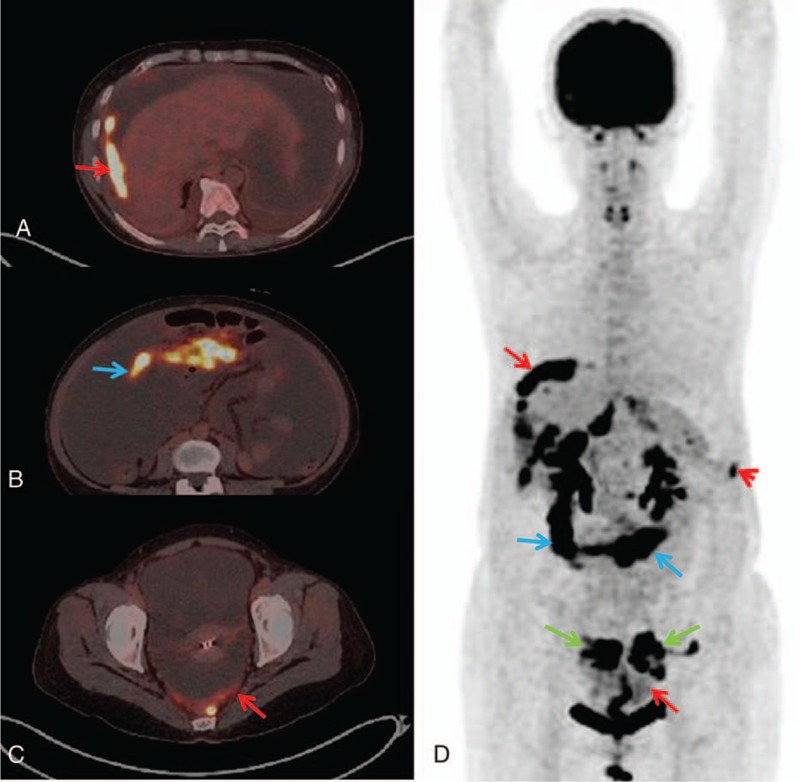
A 46-year-old woman with peritoneal carcinomatosis from ovarian cancer. Dominant distribution in the pelvic and right subdiaphragmatic region of the parietal peritoneum (the susceptible area for peritoneal implantation, SAPI) (SAPI distribution): clustered ^18^F-FDG uptake (red arrow) in the right subdiaphragmatic region (A) and the pelvic region (C) and negative findings in the bilateral paracolic gutter regions (B) on combined PET/CT axial imaging; focal ^18^F-FDG uptake (red arrowhead) in the left subdiaphragmatic region on PET maximum intensity projection (MIP) imaging (D); mass-like ^18^F-FDG uptake in the bilateral ovaries (green arrow) and the greater omentum (blue arrow) (D). CT = computed tomography, ^18^F-FDG = F18-fluorodeoxyglucose, MIP = maximum intensity projection, PET = positron emission tomography, SAPI = susceptible area for peritoneal implantation.
